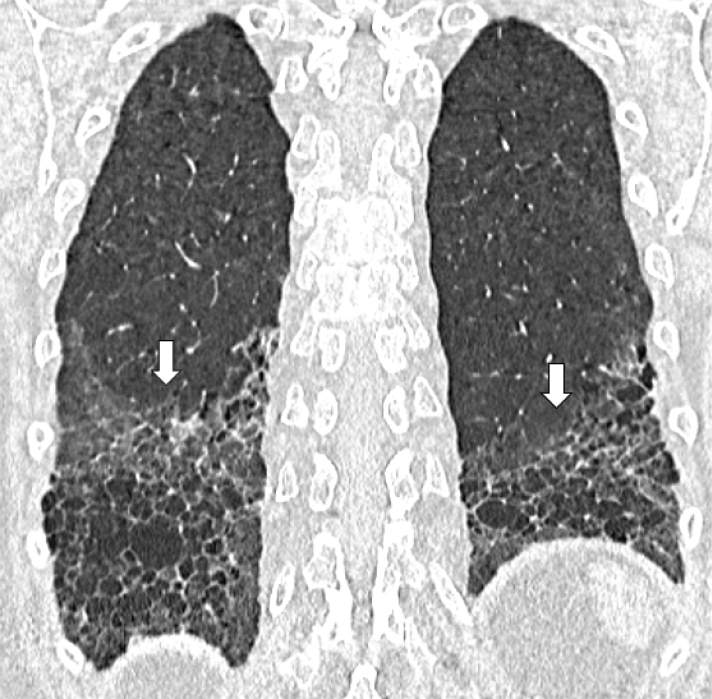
Coronal HRCT image of a case of SSc-UIP where fibrosis is predominantly located in the lung bases with a sharp and clear demarcation (arrows) in the cranio-caudal plane (“straightedge” sign). HRCT, high resolution computed tomography; SSc-UIP, systemic sclerosis-usual interstitial pneumonia.
